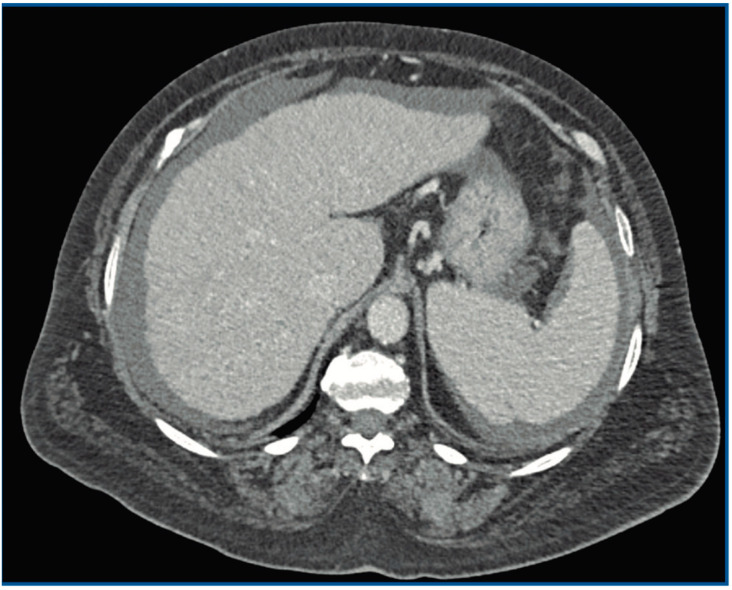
CT scan showing chronic inflammation of the liver with moderate ascites.
